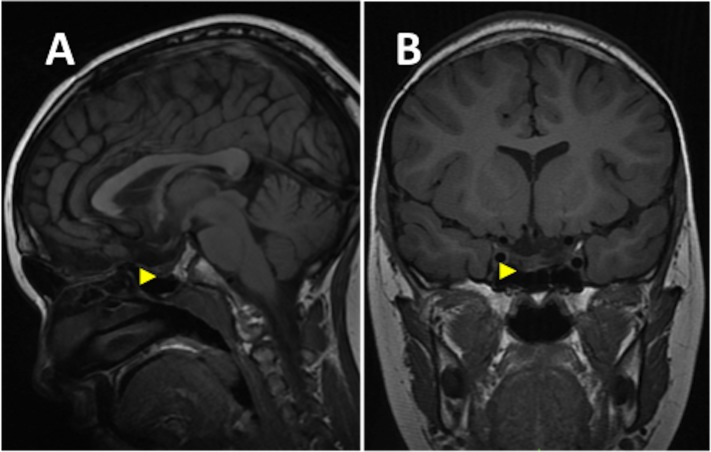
At 9 months post radiotherapy, T1-weighted FLAIR MRI brain (sagittal view [panel A], coronal view [panel B]) Shows resolution of the craniopharyngioma cyst leaving only the previously observed intrasellar residuum (arrowhead).
